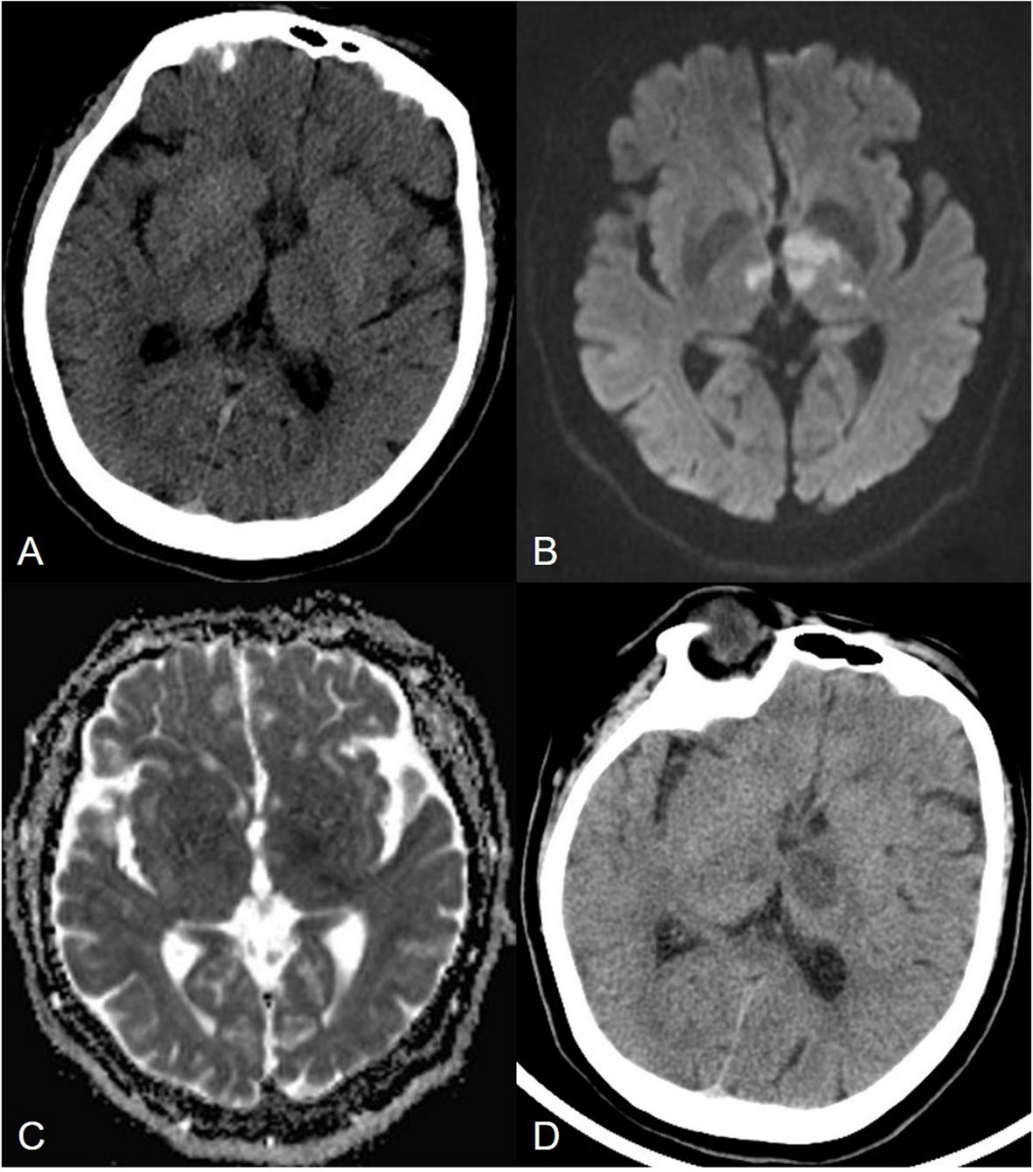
Bilateral paramedian thalamic ischemia with anterolateral paramedian territory. Case 5. Axial head CT at 2 hours after onset (A) shows no hypodensity. Axial DWI and ADC map show acute infarcts (B and C) in the bilateral paramedian thalami (white arrow) and left anterior thalami (black arrow) after 24 hours, meanwhile, head CT show hypodensity (D)
